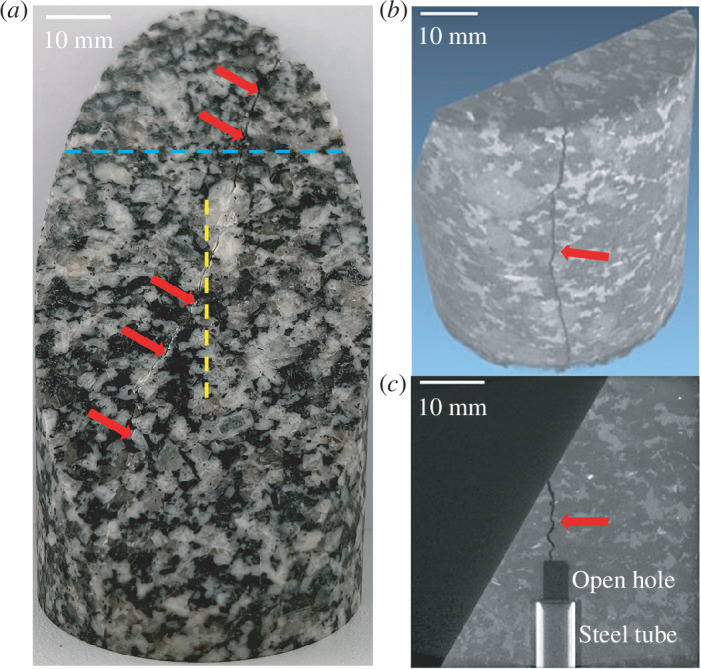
The granite sample after the fluid injection experiment where fluid was injected indirectly into a fault. (*a*) Fault surface of the lower sample half after the experiment. The blue dashed line indicates the upper bound of the CT image in (*b*). The yellow dashed line represents the position of the section in (*c*). The red arrows indicate the fracture induced by fluid injection during the experiment. (*b*) The three-dimensional digitally reconstructed sample based on the CT images. (*c*) The CT image of a cross-section of the lower sample half.
